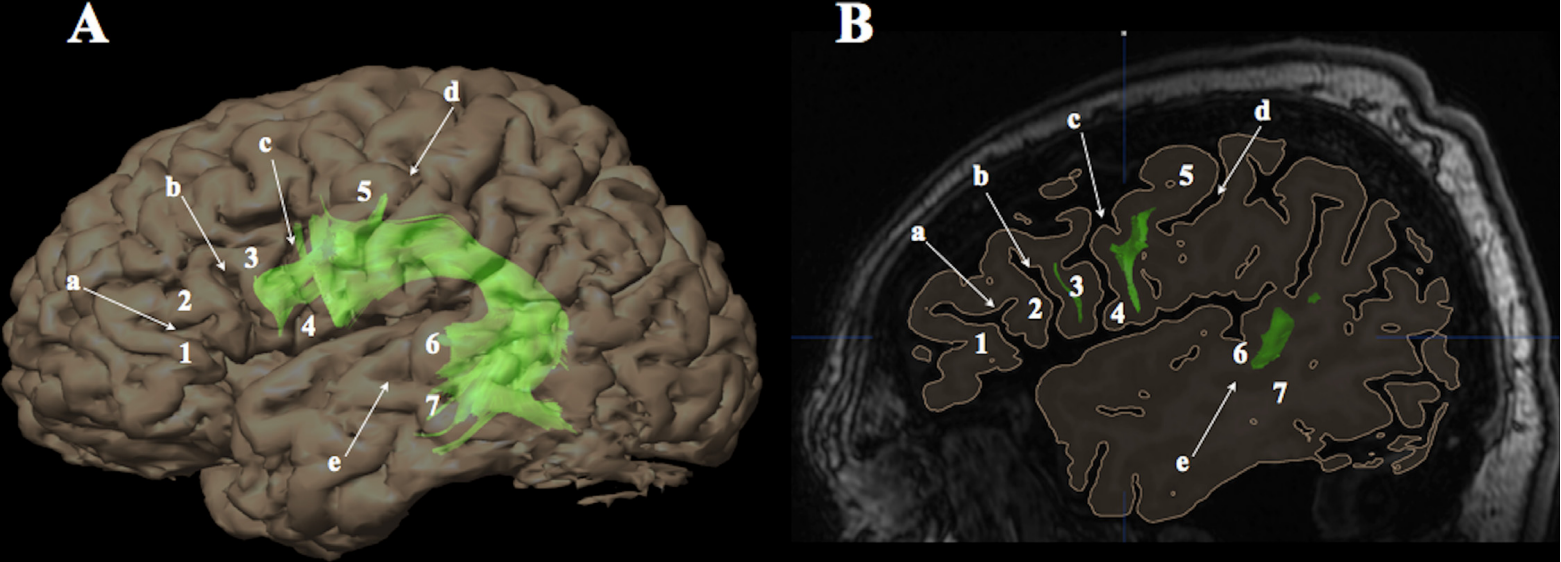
Labeling of cortical terminations according to anatomical landmarks. Cortical terminations of white matter fascicles (e.g. left arcuate fascicle; green) were labeled according to gyri and sulci provided by 3D surface renderings (A) generated from high-resolution T1-weighted magnetic resonance images (B; sagittal section running through the left frontal operculum). a = horizontal ramus of the Sylvian fissure; b = ascending ramus of the Sylvian fissure; c = precentral sulcus; d = central sulcus; e = superior temporal sulcus; 1 = pars orbitalis of the inferior frontal gyrus (Brodmann area [BA] 47); 2 = pars triangularis (BA 45); 3 = pars opercularis (BA 44); 4 = ventral premotor cortex (BA 6); 5 = precentral gyrus (BA 4/6); 6 = superior temporal gyrus (BA 22); 7 = middle temporal gyrus (BA 21).
